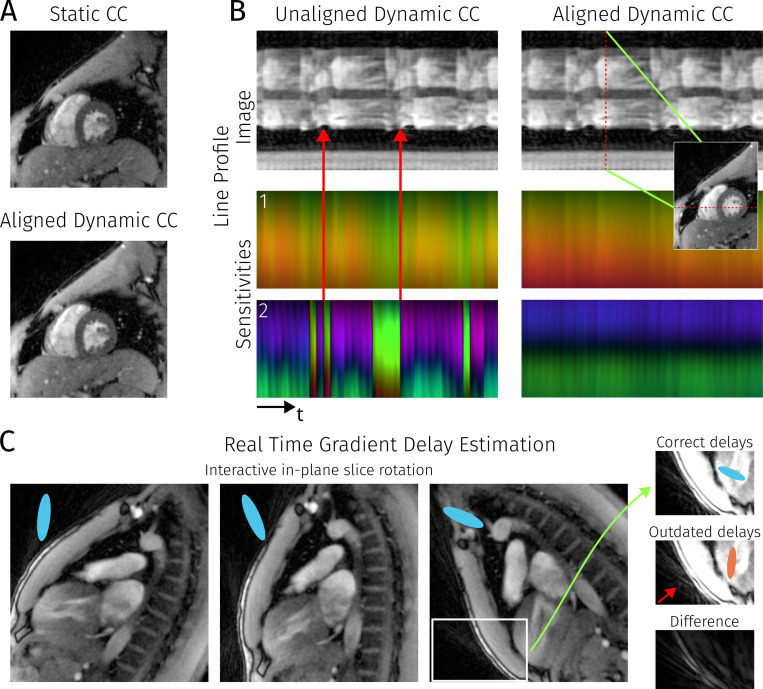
Part A compares two frames from a RT-MRI of the heart with different strategies for coil compression, either re-using the compressing matrix or recomputing and aligning it for every frame. The red arrows highlight abrupt changes in image magnitude which correlate with the discontinuities in the virtual coil profiles. Part B shows a time series from a line through the heart, with the line being shown in the small inset image. The bottom row shows the time series for the first two virtual coil profiles, the top row shows the actual images. On the left, every frame was independently compressed, on the right, compression matrices were aligned using geometric coil compression. Part C highlights a different aspect of preprocessing, showing three frames from an interactive real-time MRI session where the in-plane rotation was changed over time. Estimated gradient delays are visualized using ellipses shown in the top left corner of the respective images. A zoom-in on the right shows two reconstructions of the last frame, using either the current gradient delay estimation (top) or gradient delay estimations from the first frame (center), resulting in increased artefacts (red arrow).
